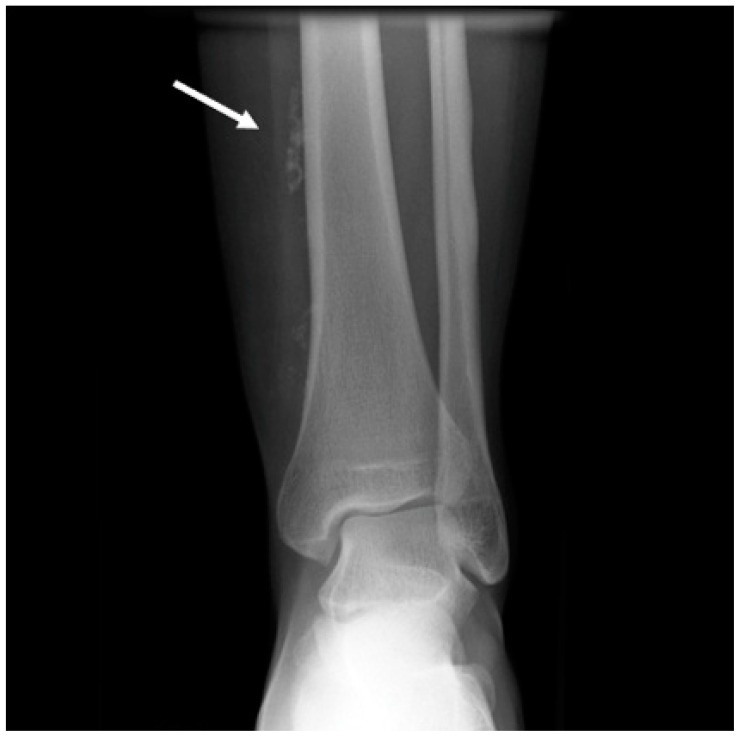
Anteroposterior X-rays showing phlebolites (white arrow) within soft tissues near the tibial diaphysis.
